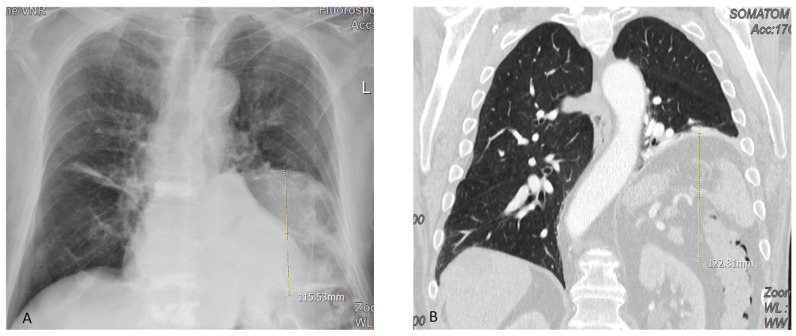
Right diaphragmatic eventration on the chest X-ray (A) and CT scan (B). The yellow line on (A) and (B) is an example of one patient’s actual length of diaphragmatic displacement.
